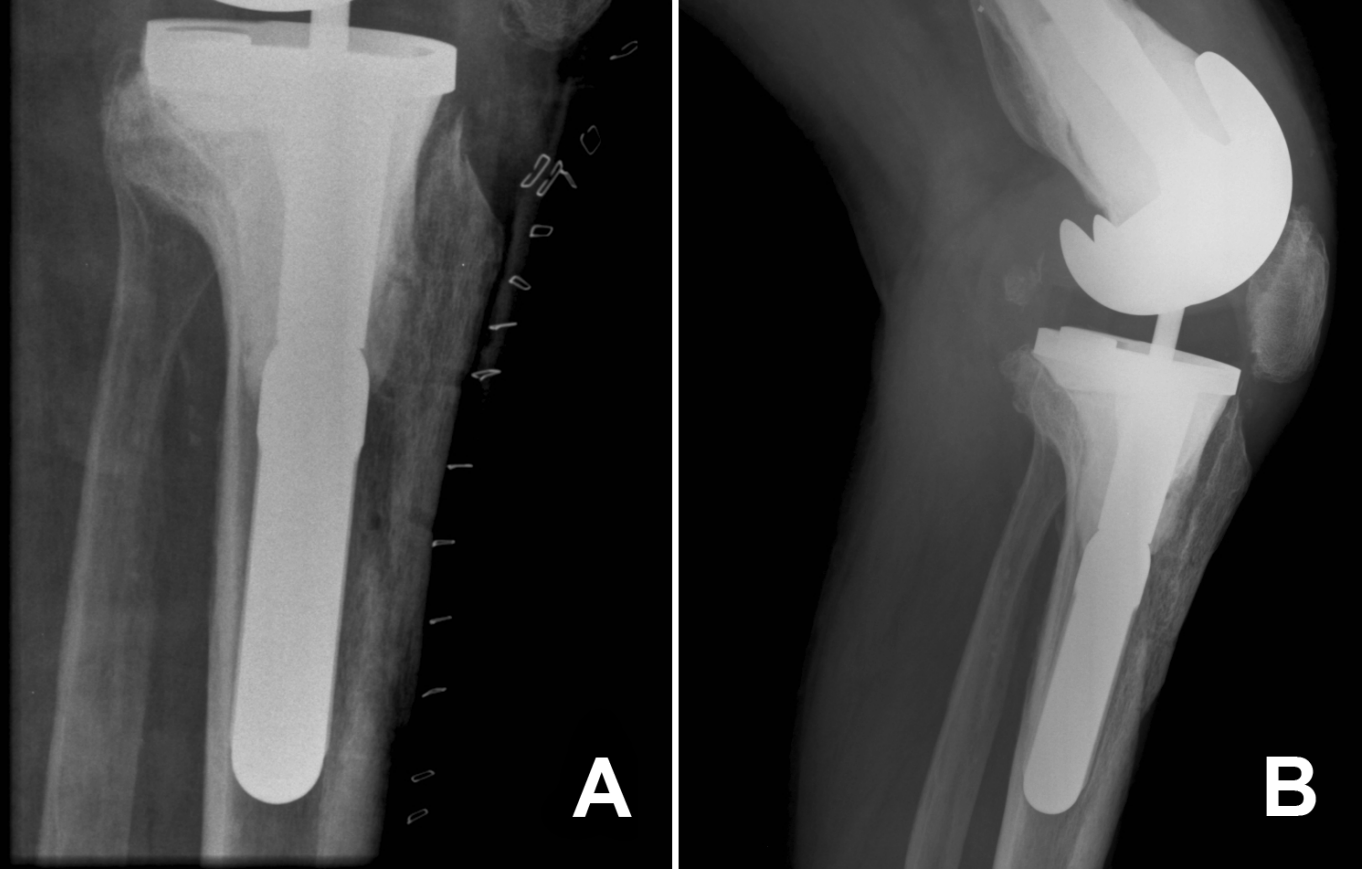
Radiograph of suture repair of tibial tubercle osteotomy immediately post op (A) and at follow-up demonstrating trabeculae crossing the osteotomy site (B).
